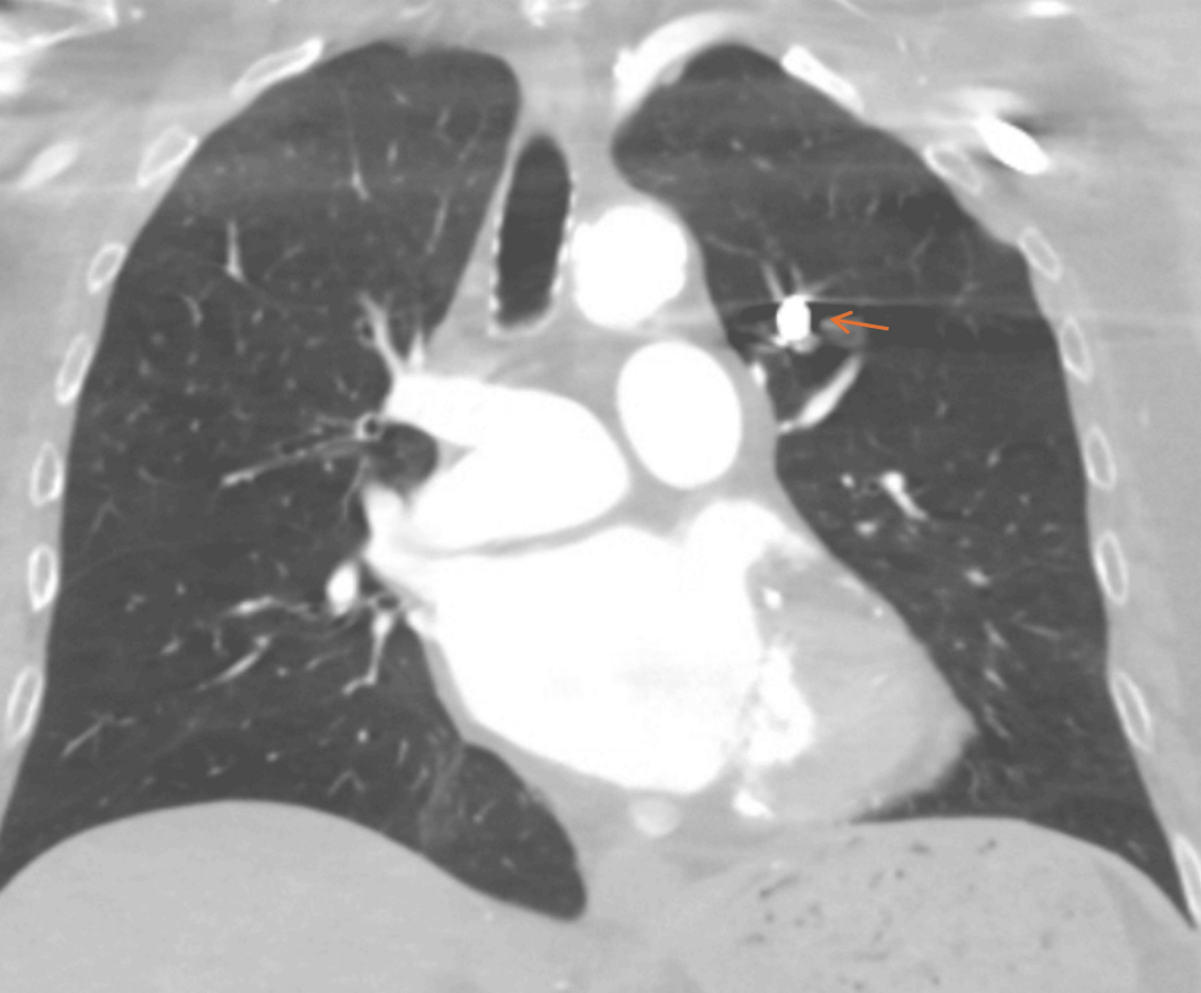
Coronal CT scan showing the same retained metallic object in the left pulmonary artery consistent with a retained bullet (orange arrow).
